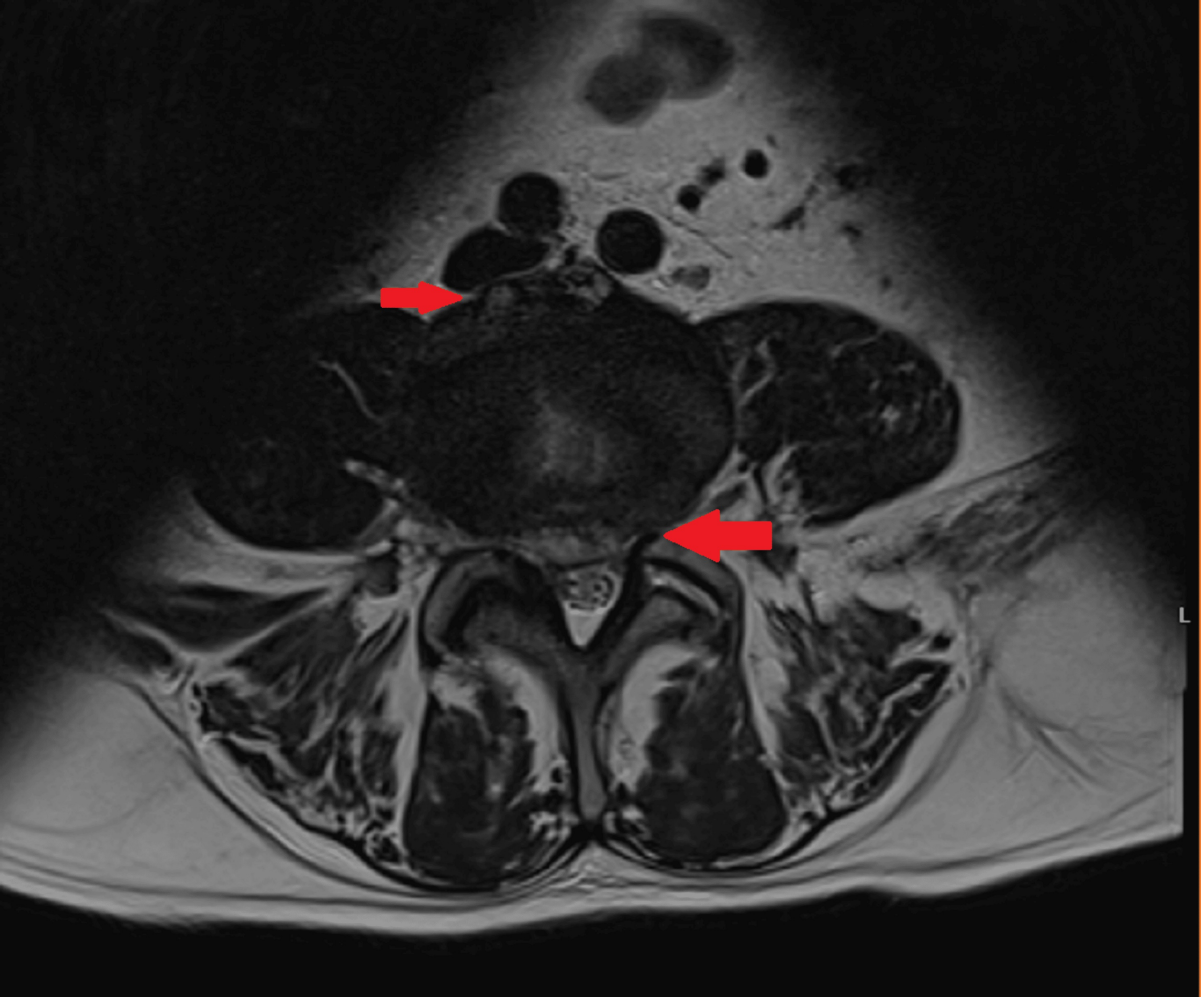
Initial lumbosacral MRI (T2, transversal) showing edema in the L4 and L5 vertebral bodies and an epidural abscess
